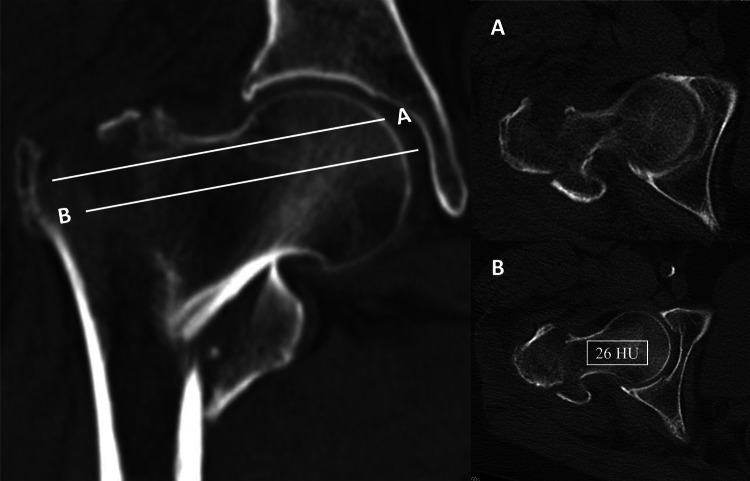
Methodology for measuring proximal femoral CT Hounsfield units: (A) reference axial slice (first slice; 0 mm); (B) targeted axial slice (second slice; 5 mm). This patient’s proximal femoral CT Hounsfield unit was 26 HU, and she eventually experienced varus deformity and delayed nonunion
